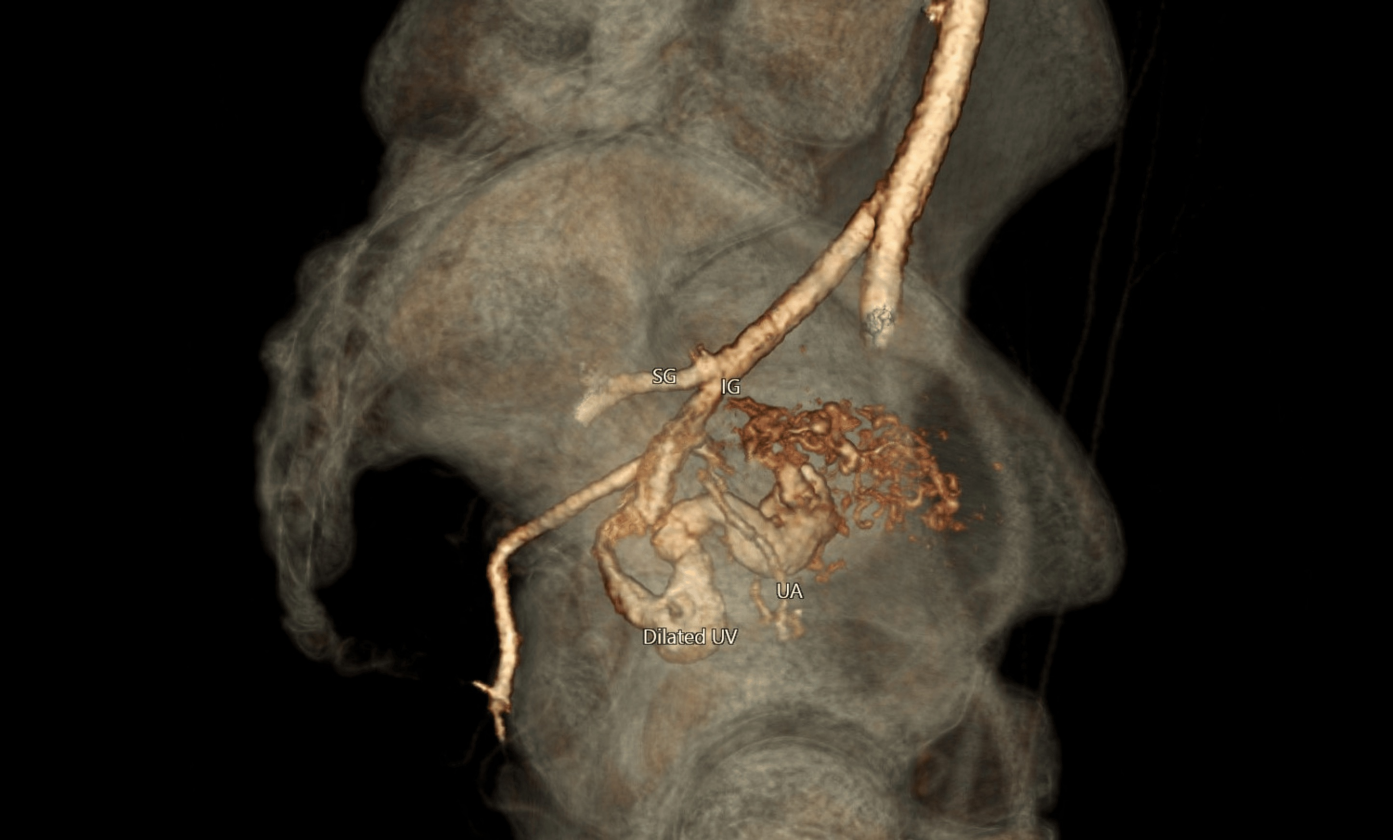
Three-dimensional reconstruction of CT angiogram demonstrating the grossly dilated uterine vein as it flows from the arteriovenous malformation. SG = superior gluteal artery; IG = inferior gluteal artery; UA = uterine artery; UV = uterine vein
